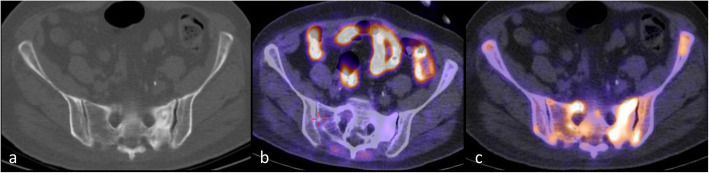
Comparison of NaF and FDG PET-CT In the same 65-year-old woman with metastatic breast cancer a CT (a) shows increased sclerosis in the left sacrum, increased tracer uptake of NaF PET in left sacrum and also in both ilium (b) but disease activity less well seen on FDG PET (c)
